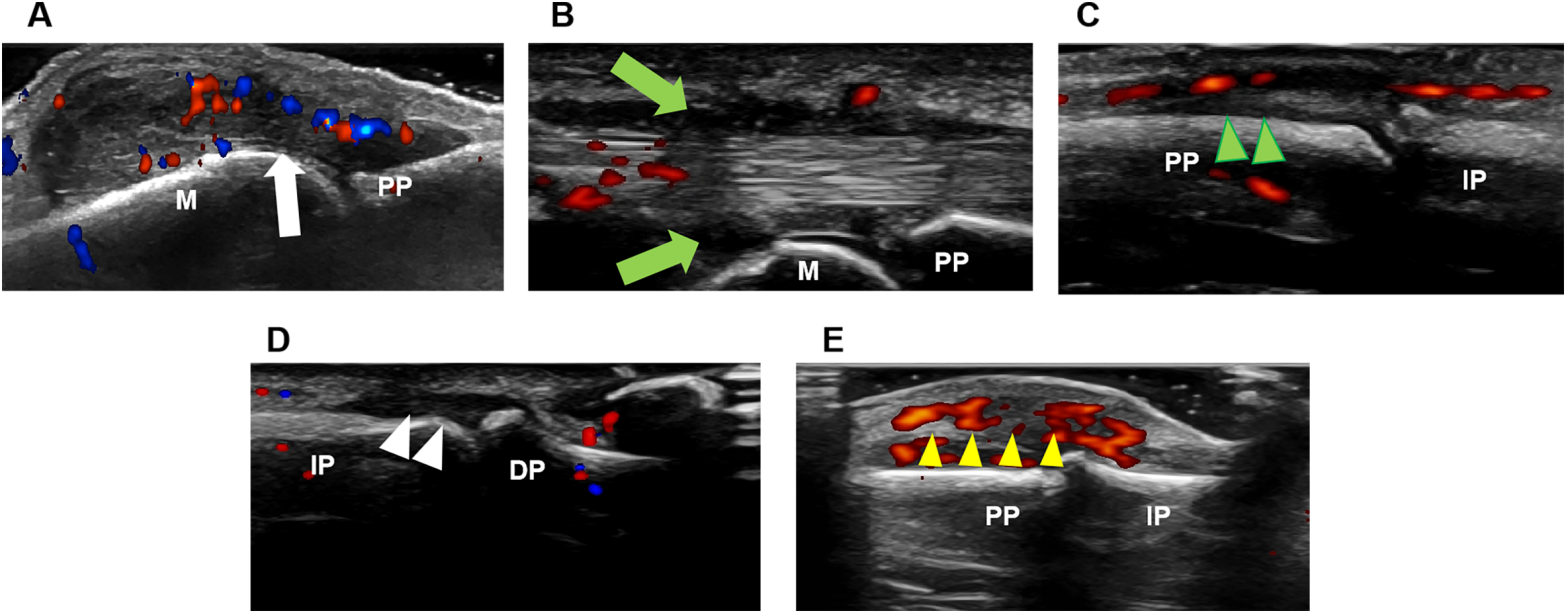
Ultrasound imaging of diverse inflammatory lesions in the hand. (A) Synovitis (white arrow). (B) Tenosynovitis (green arrow). (C) Paratenonitis (green arrowhead). (D) Enthesitis (white arrowhead). (E) Soft tissue inflammation (yellow arrowhead). DP, distal phalanx; IP, intermediate phalanx; M, metacarpal bone; PP, proximal phalanx.
